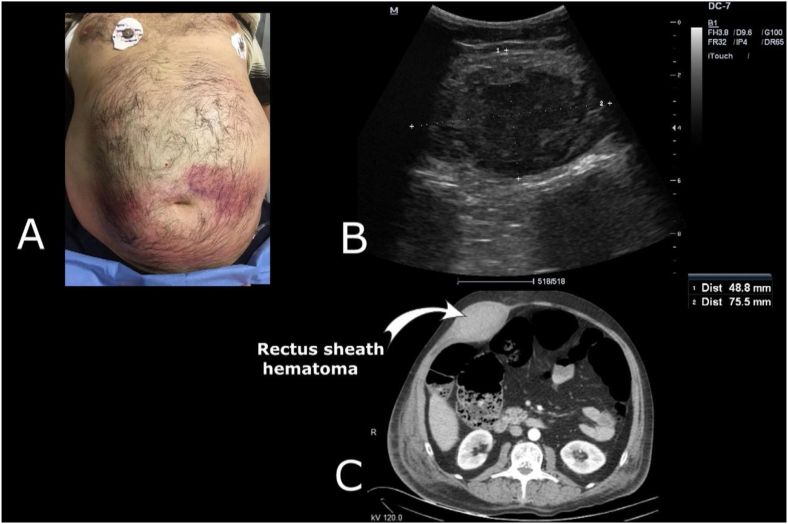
Physical examination finding, ultrasound image, and CT image of the patient with rectus sheath hematoma. A, Redness and ecchymotic areas of abdominal skin that occurred within minutes after abdominal pain; B, Ultrasonographic measurement of the diameter of rectus sheath hematoma with curvilinear probe; C, Computed tomography image showing rectus sheath hematoma that did not invade into the intra-abdominal area.
